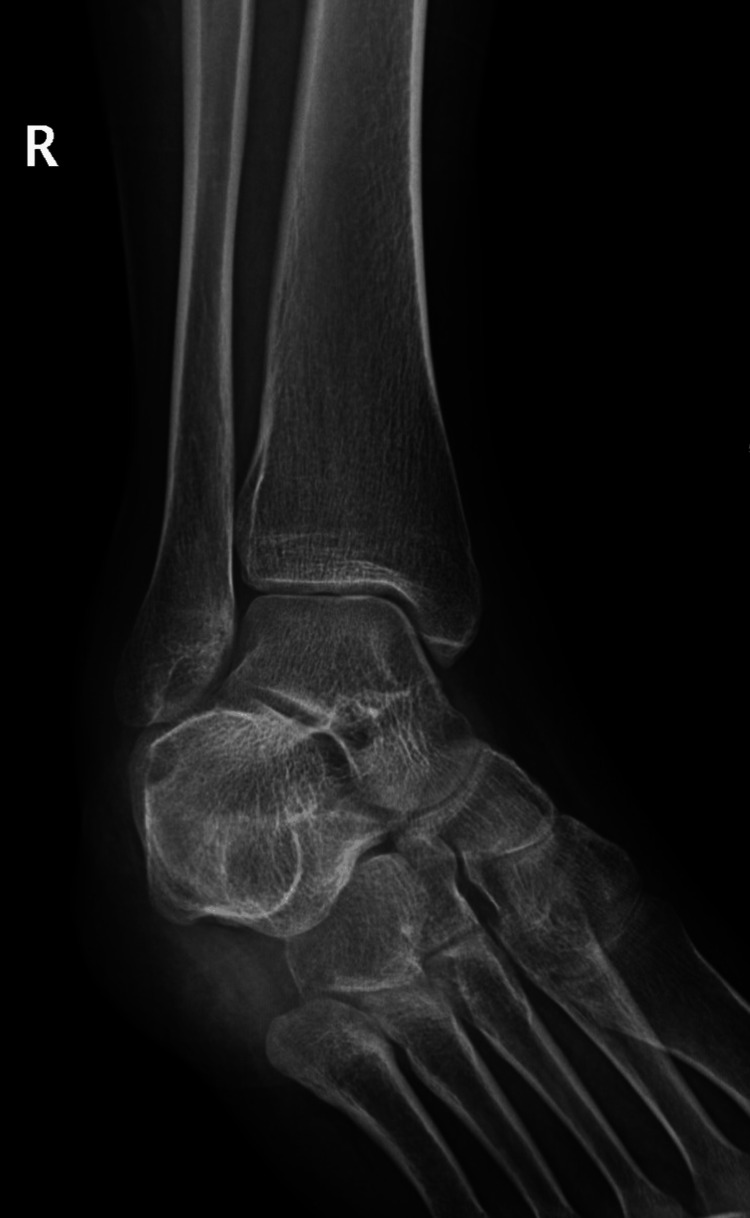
Mortise view X-ray of the right ankle at the time of presentation
